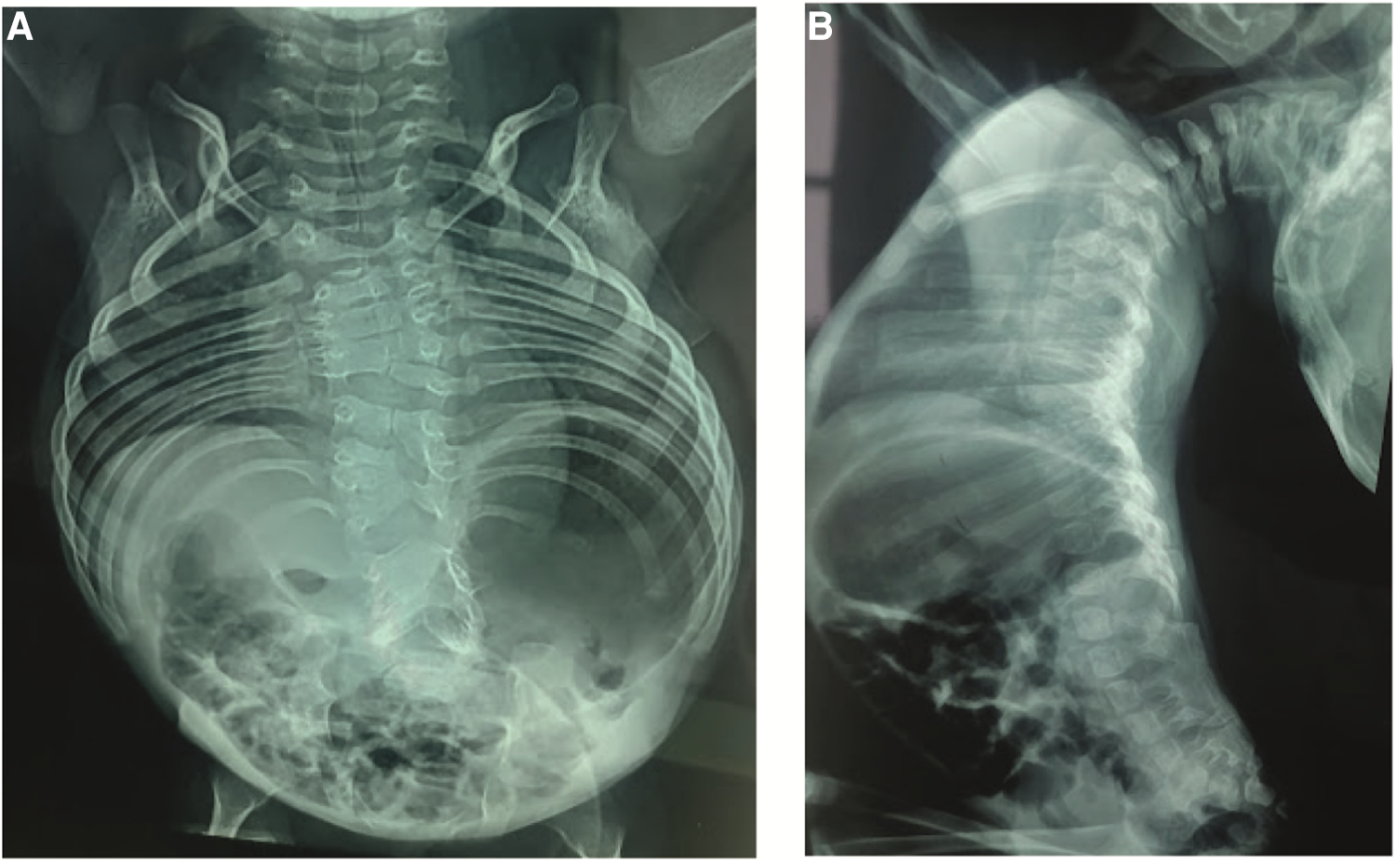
(A) Anteroposterior x-rays view showing the multiple vertebral defects and the “crab-like” aspect of the rib cage. (B) Lateral view showing the hyperlordosis and the fusion of the spinal processes.
